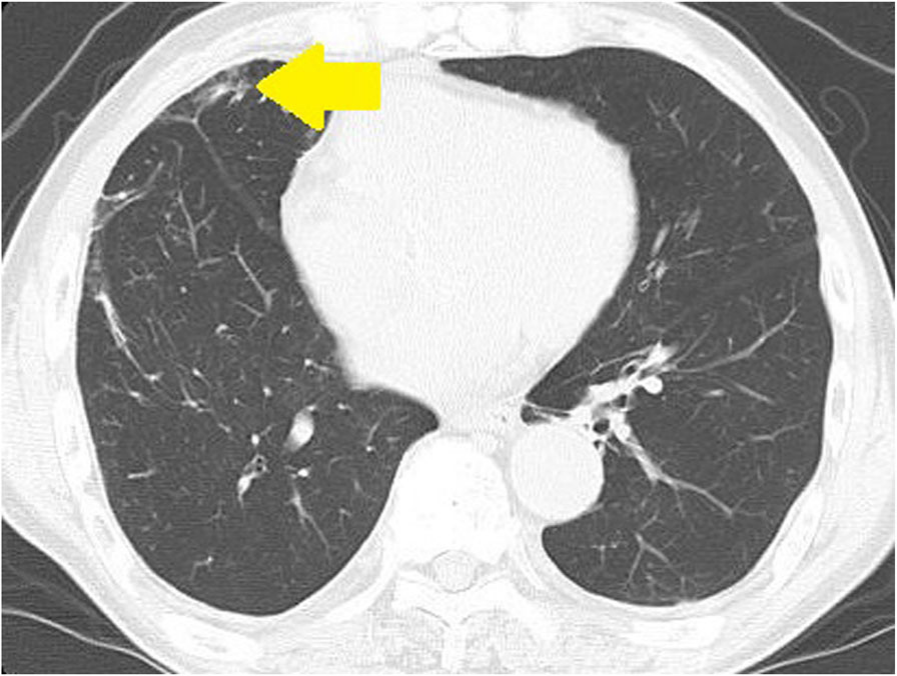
Chest computed tomography. a 12-mm right lower lobe nodule with ground glass opacity
